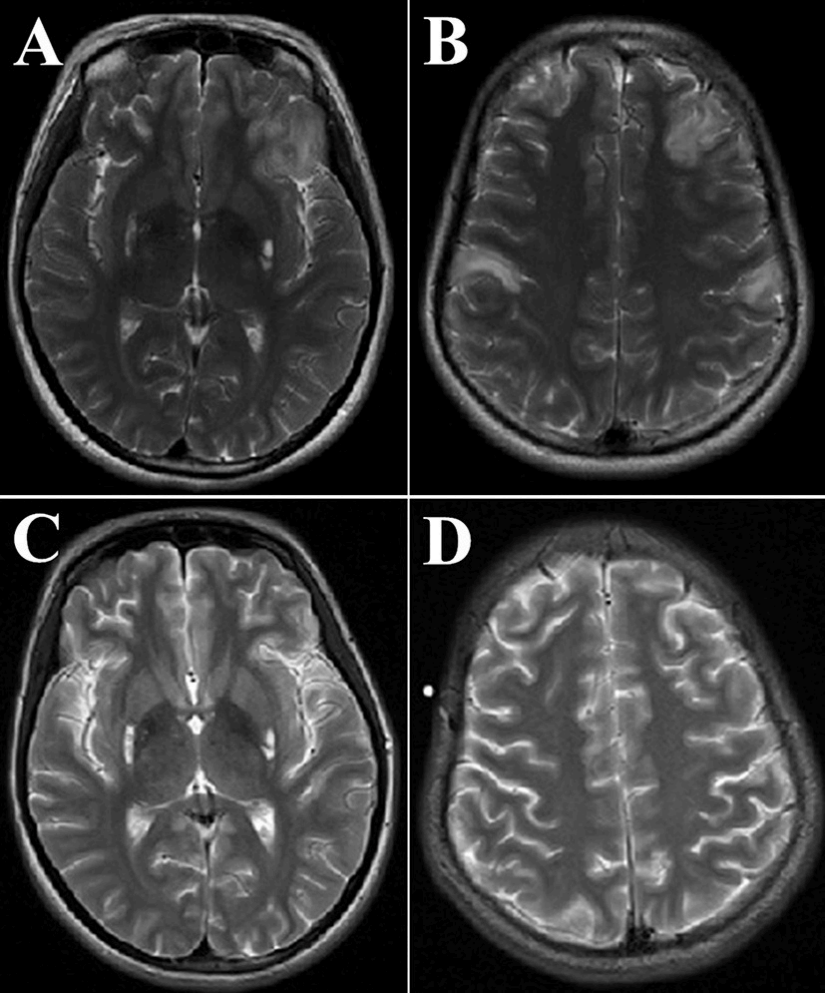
The proband's brain magnetic resonance imaging (MRI) obtained at 24 years old. Axial T2-weighted images of the brain MRI were obtained at the emergency department [Panels (A) and (B), respectively], 24 days after treatment, the brain MRI was redone [Panels (C) and (D), respectively]. Panels (A,B) showed frontal and parietal lobes cortical lesions and bilateral high-intensity areas in the putamen. Panels (C,D) showed that hyper signals in T2-weighted images of symmetrical putamen did not change significantly; however, the cortical lesions obviously improved.
